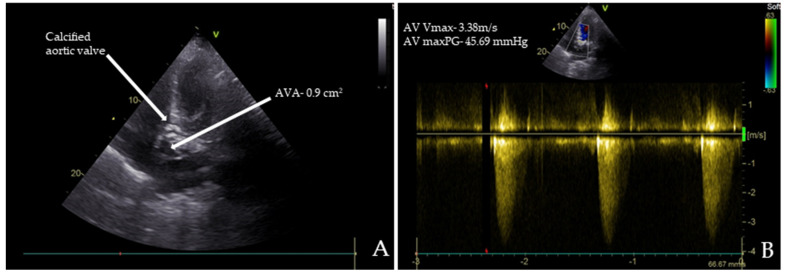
Transthoracic echocardiography (TTE) images of the patient: (A) TTE reveals severe aortic stenosis with a calcified aortic valve (AV) and an aortic valve area (AVA) of 0.90 cm²; (B) the maximum peak gradients (AV maxPG) across the AV were 45.69 mmHg, and the maximum systolic flow velocities (AV Vmax) were 3.38 m/s.
